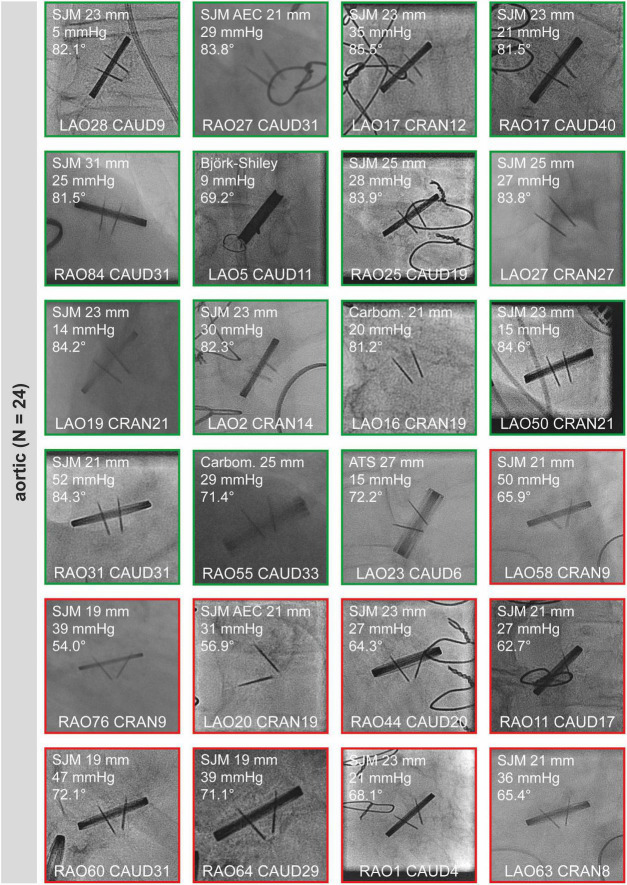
Sufficient cinefluoroscopy studies of MHVs in aortic position. MHVs had either normal leaflet opening and closing (green) or leaflet dysfunction (red). MHVs are shown during maximum opening. Individual images show the opening angle (for details refer to Figure 1), mean gradient from transthoracic echocardiography, and projection angles in CF. For comparison, the mean opening angles of SJM, ATS, and Carbomedics MHVs are 83.4, 69.5, and 77.1°, respectively (12). Some images are already shown in Figure 1. AEC, aortic valve extended cuff; ATS, ATS Medical; Carbom., Carbomedics; CAUD, caudal; CRAN, cranial; LAO, left anterior oblique; MHV, mechanical heart valve; RAO, right anterior oblique; SJM, St. Jude Medical.
